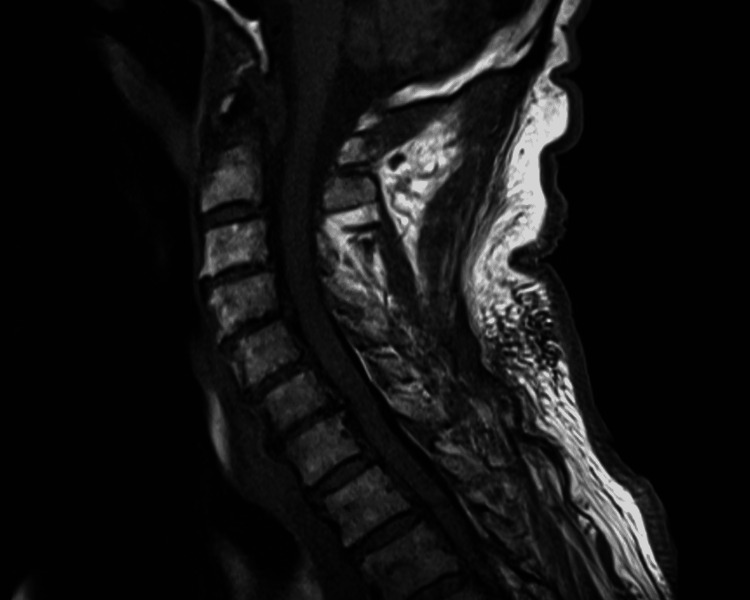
MRI of the cervical spine, showing multilevel degenerative disc disease and no signs of demyelination, fracture deformity, traumatic subluxation, or compressive myelopathy
